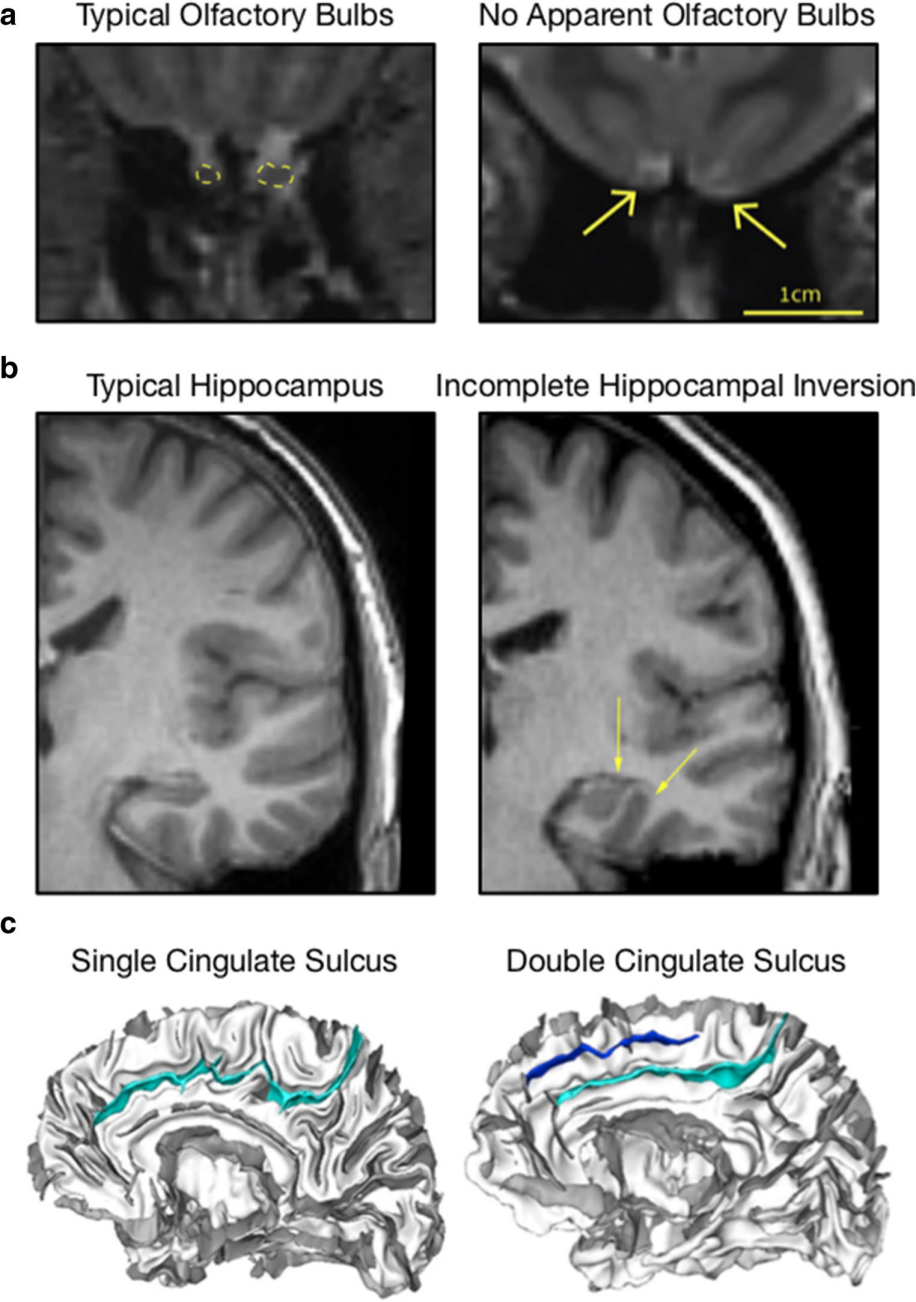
Examples of infrequent morphological features examined in large datasets. a Typical olfactory bulbs and no apparent bulbs in monozygotic twins, shown on a T2-weighted coronal image, adapted from Weiss et al. (2020). b Typical hippocampus and incomplete hippocampal inversion, shown on a T1-weighted coronal image, adapted from Caciagli et al. (2019). c Single and double cingulate sulcus, shown on the medial view of a reconstructed cortical surface, adapted from Cachia et al. (2016).
